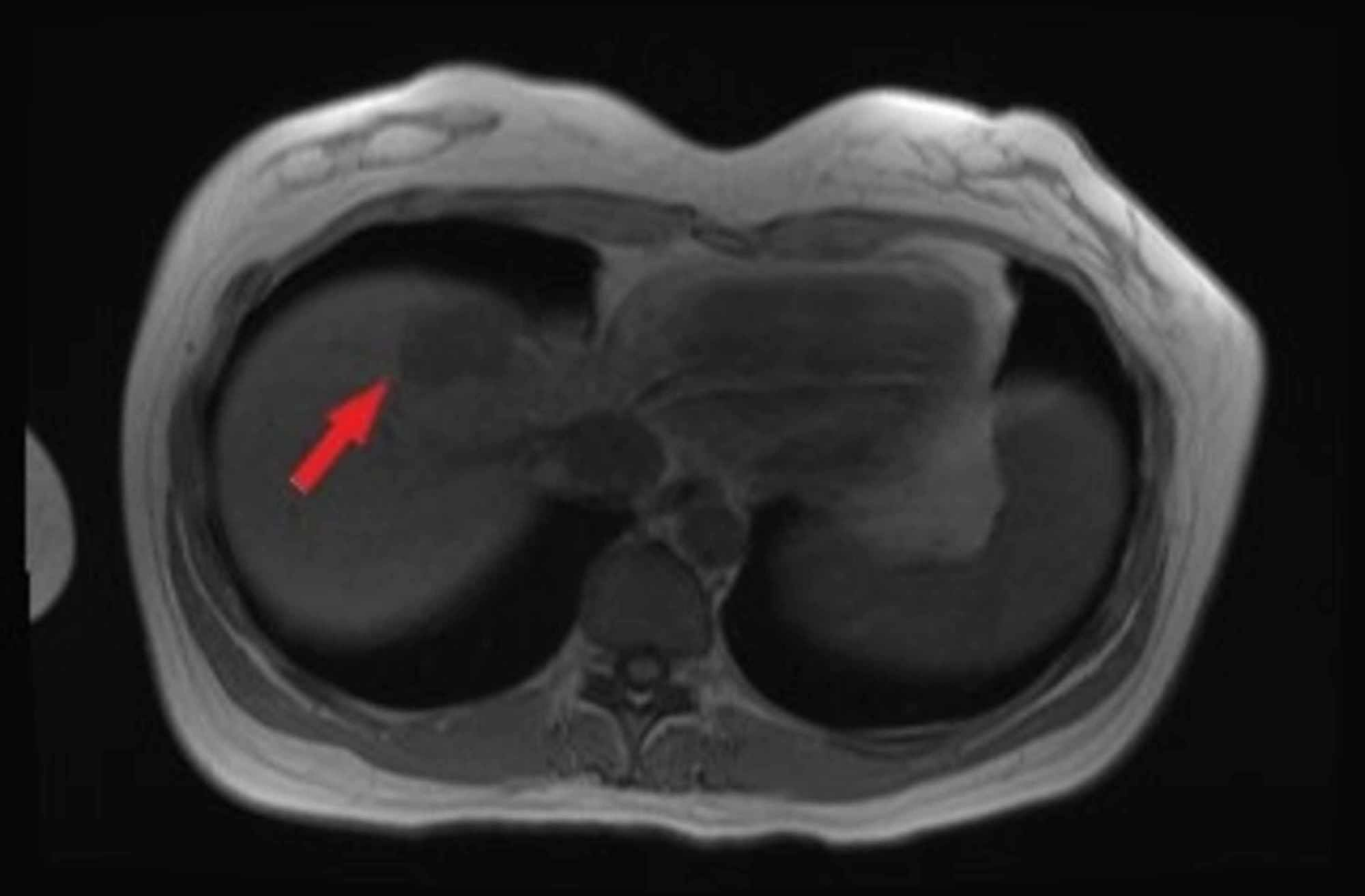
MRI Mass corresponds with hypo-attenuating lesion seen on CT scan.
